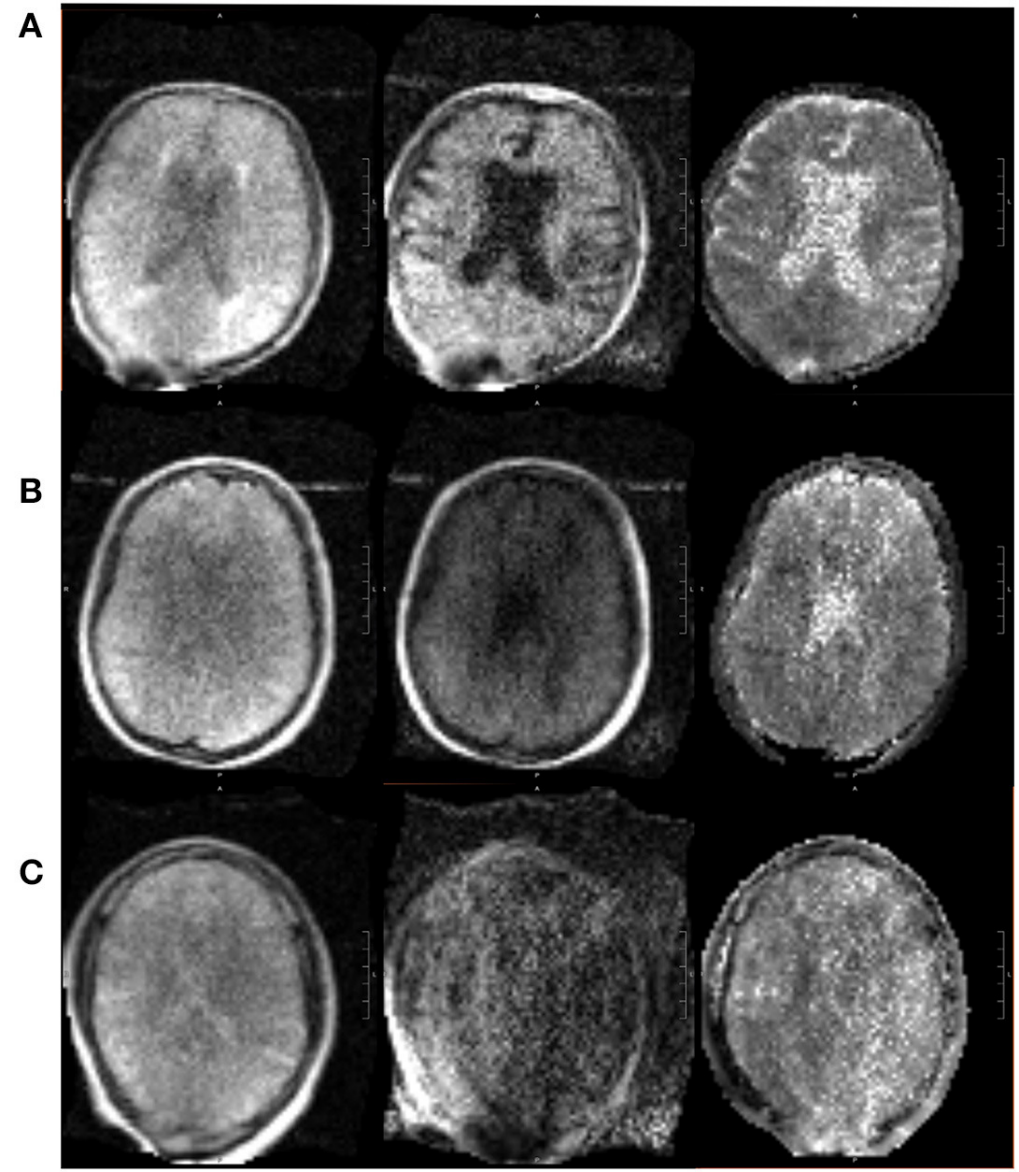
Range in pMRI DWI quality. Three sample DWI sequences are provided, of varying quality. From left to right: DWI (b = 0), DWI, DWI with ADC map. (A) In a relatively good scan, the sulci are appreciable, ventricles are distinct, and gray vs. white matter can be distinguished. (B) In an average scan, the sulci are less visible, and the ventricles are present but not clearly delineated. (C) In a poor scan, there is significant noise and pixelation such that structures cannot be adequately identified.
